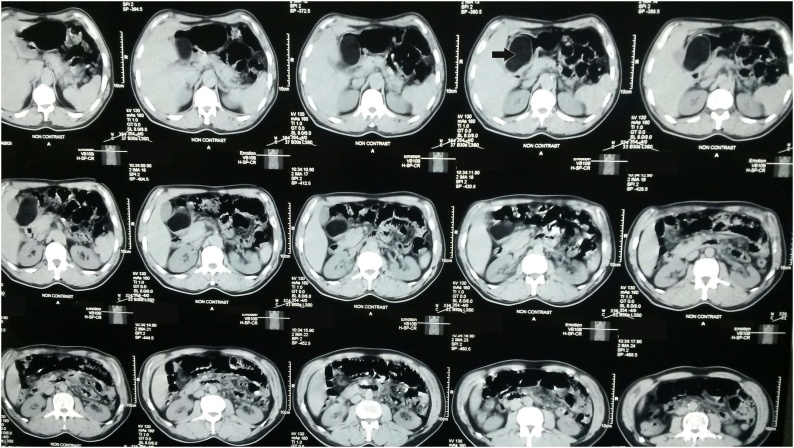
Abdominal CT shows a 4.5 × 8 cm^2^ submucosal encapsulated mass in the lower pyloric partition, fatty in its nature.
